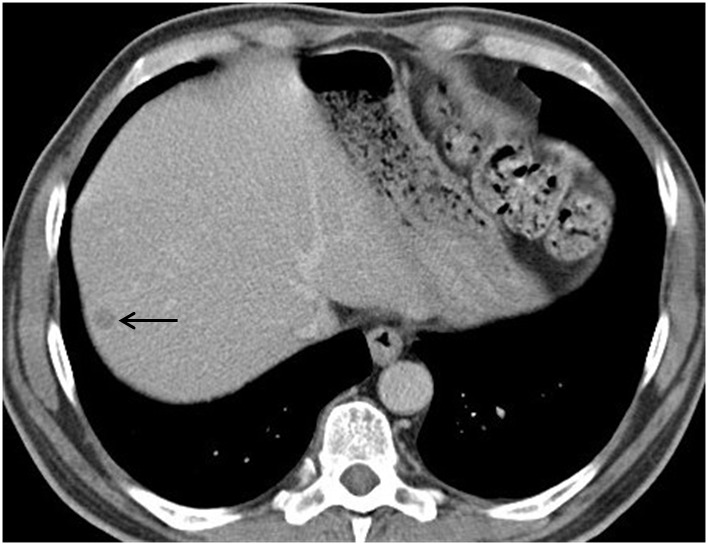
Contrast enhanced portal venous phase computed tomography demonstrating 2cm subcapsular lesion in segment 7 of the liver. The lesion displays typical low attenuation of a soft tissue mass characteristic of a colorectal liver metastasis.
